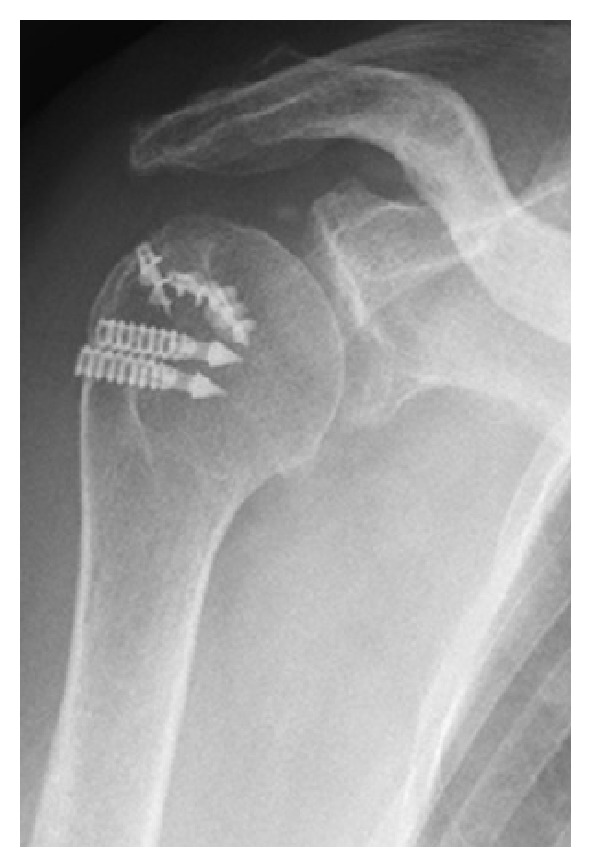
X-ray imaging after arthroscopic rotator cuff repair using a suture bridge technique.
